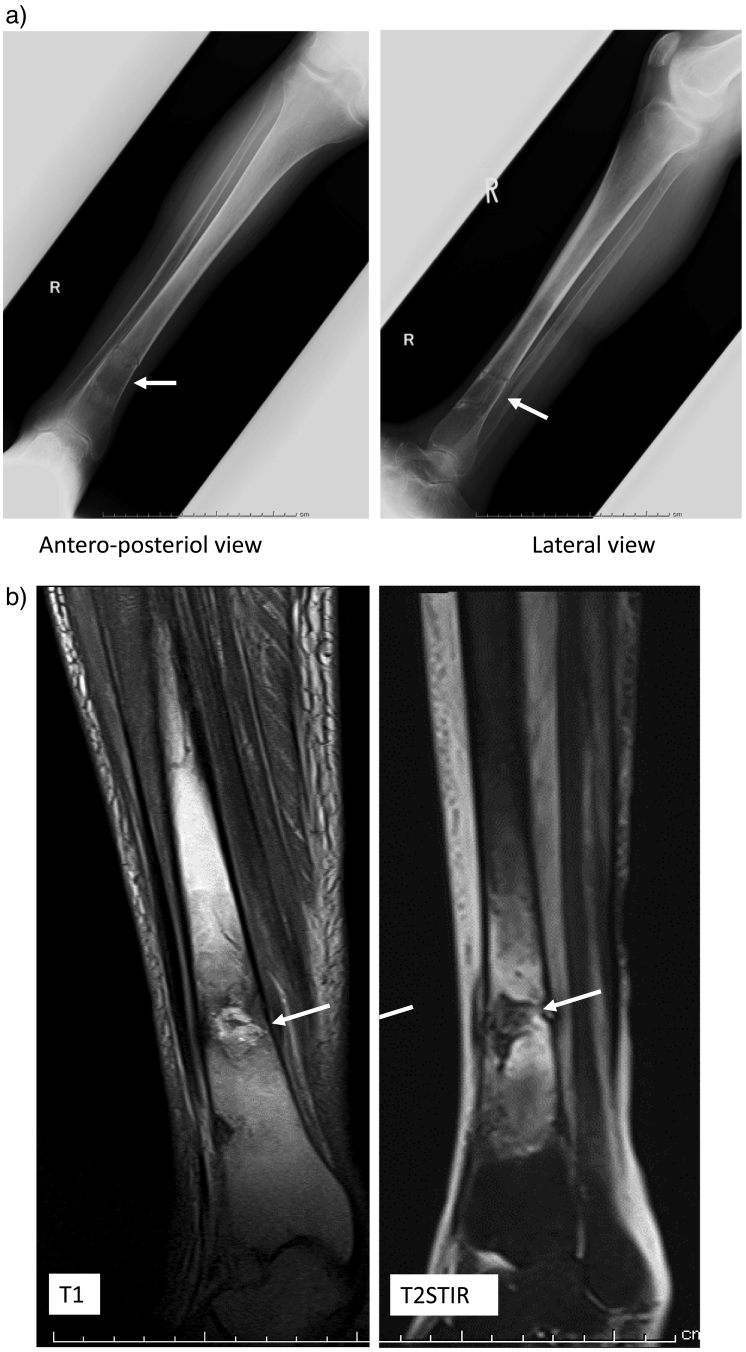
a: Plain radiograph shows a radiolucent lesion associated with a fracture line in the distal right tibia. b: Magnetic resonance imaging reveals a bone tumor-like lesion and fracture line in the right tibia. MRI T1-weighted images shows hyper intensity, T2-weighted images shows hypo intensity, and T2STIR(short-tI inversion recovery) shows hypo intensity, but was inconsistent with malignant bone tumor pattern that T1 shows low intensity to iso intensity, and T2 shows high intensity to iso intensiy.
